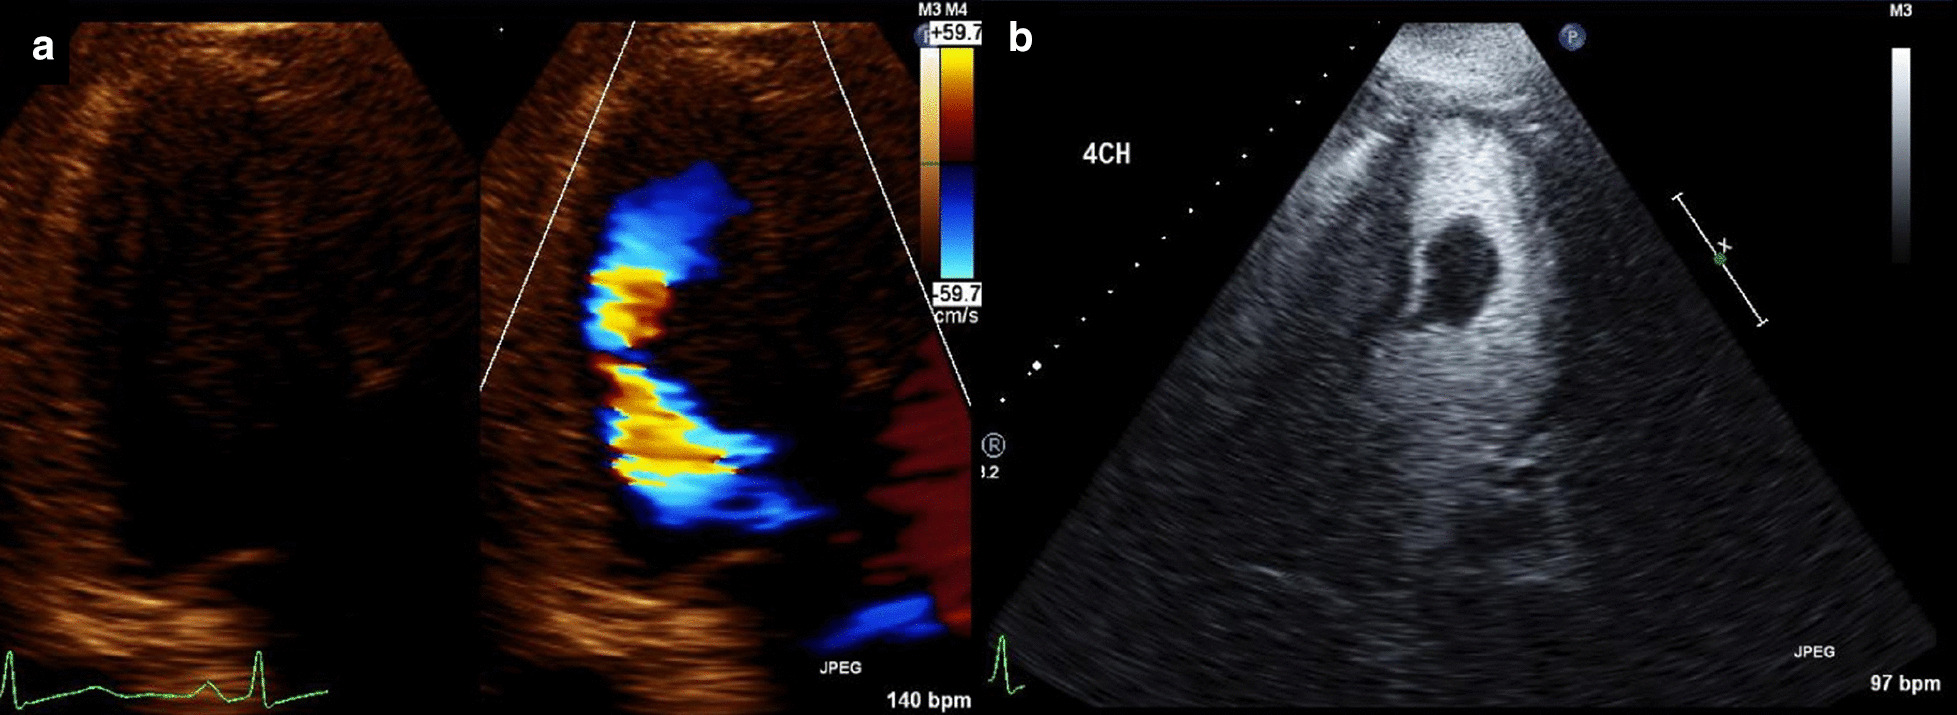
Case 3. Transthoracic echocardiography (TTE) apical LV views with and without ultrasound enhancing agent
